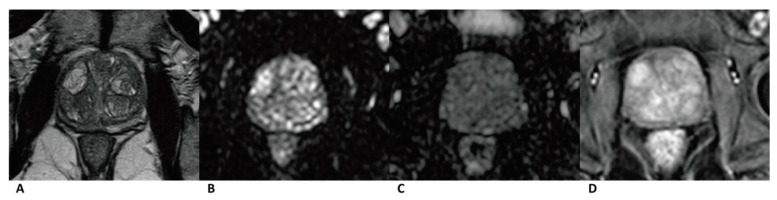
Case of benign prostate hyperplasia. Male, 64 years old, benign prostate hyperplasia. T2WI showed multiple slightly hypo/hyper-intense encapsulated nodules in TZ (A). The lesion was isointense on the DWI and ADC map (B,C). A diffuse popcorn-like enhancement was seen on the DCE and was assessed as negative (D). It was finally assessed as a category 2, indicating that it had a low cancer risk according to PI-RADS v2, pa PI-RADS v2, and biparametric MRI protocols.
